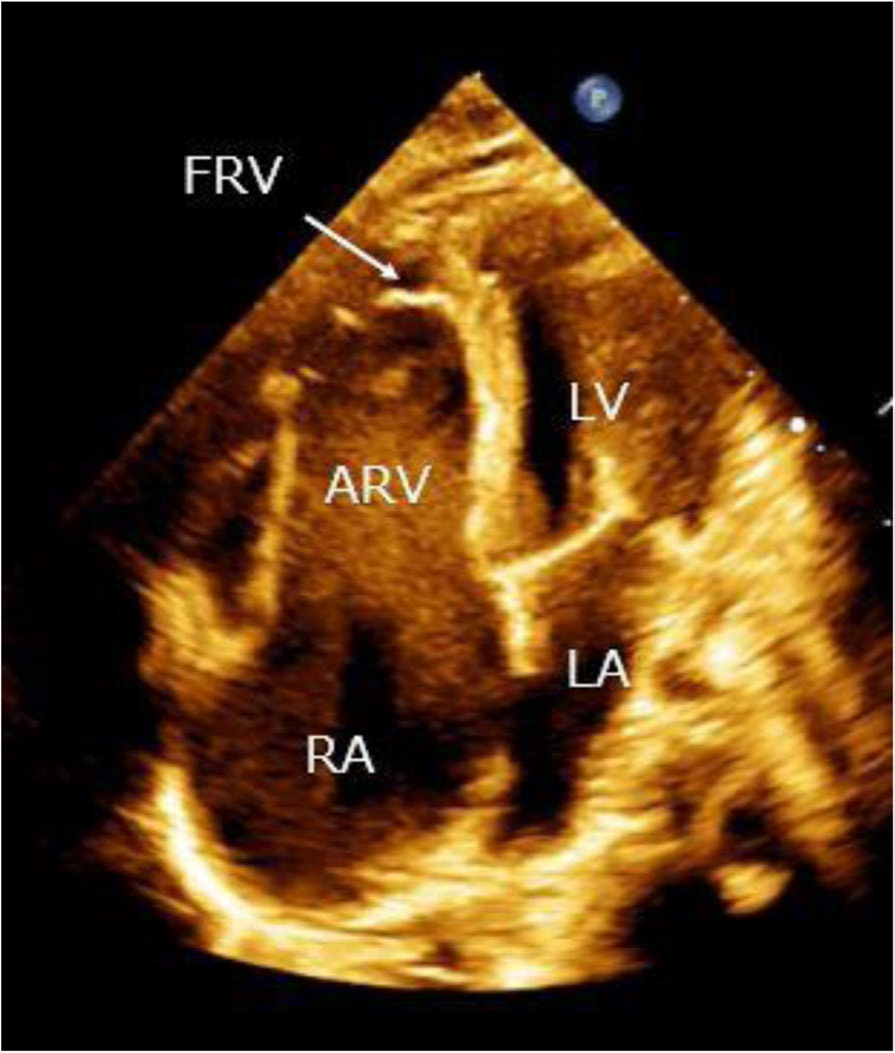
An apical four-chamber view of trans-thoracic echocardiography. RA, right atrium; LA, left atrium; ARV, atrialized right ventricle; FRV, functional right ventricle
